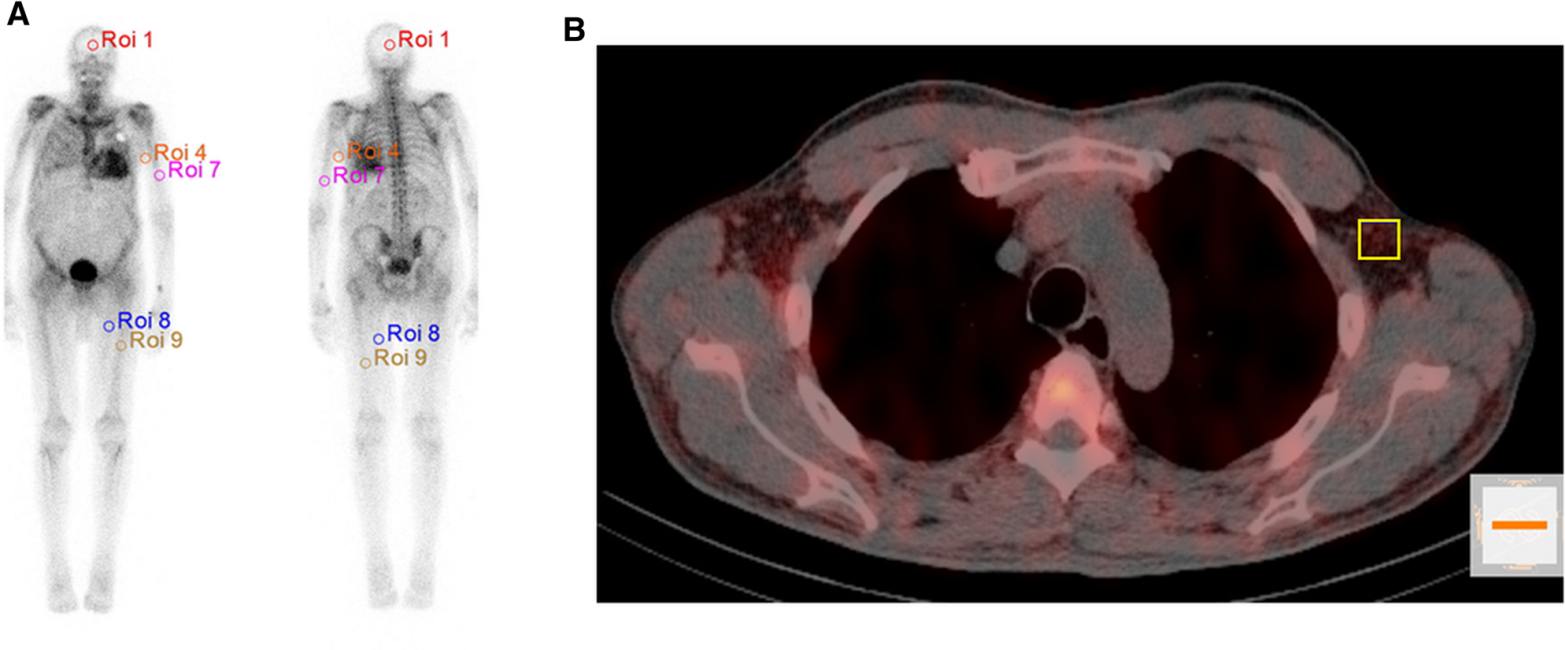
Evaluation methodology of DPD bone scintigraphy for cardiac amyloidosis. (a) Showing the ROI localization for the skull uptake (red ROI1) as well as the ratio of soft tissue to humeral shaft (orange ROI 4 and pink ROI 7) and the soft tissue to femoral shaft estimation (blue ROI 8 and brown ROI 9) and (b) for the soft tissue tracer uptake of subcutaneous fat in the left axillar region
